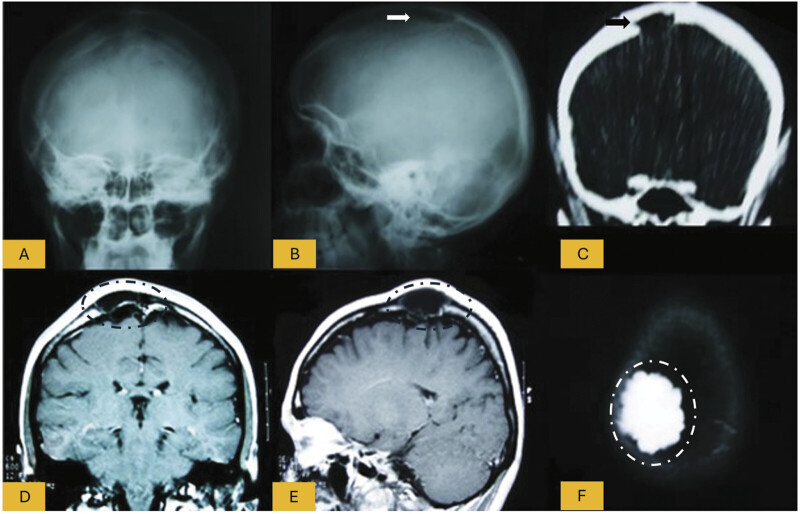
AP and lateral skull radiographies show a well-defined expansile lytic lesion located in the intradiploic region of the right parietal bone adjacent to the vertex region (white arrow) (
